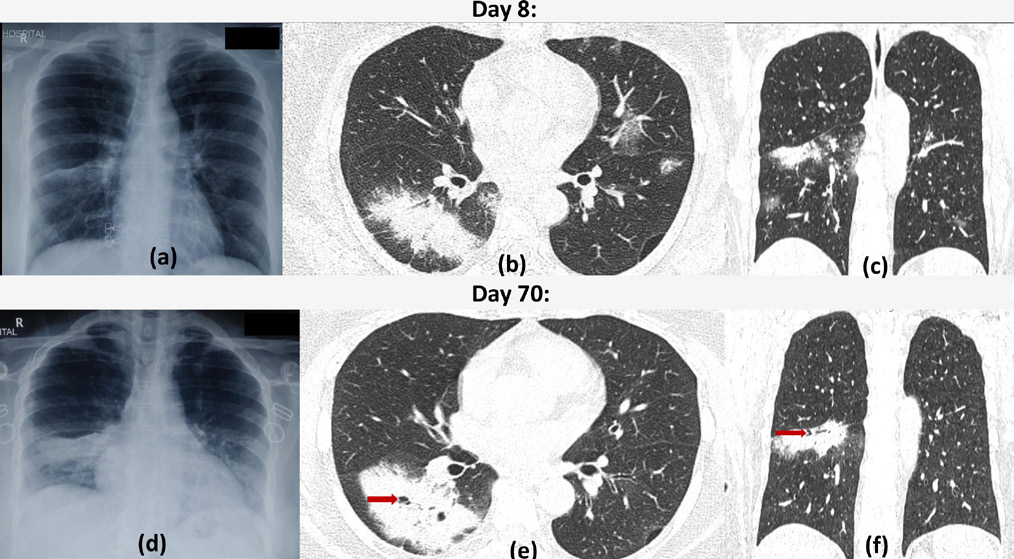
Cavitation within consolidation as a sequelae to COVID19 infection: 52-year-old female with fever tested positive for COVID19 infection, no respiratory complaints. Frontal chest radiograph (a) on the eighth day of illness shows a patchy opacity in the right mid zone. Chest HRCT (axial and coronal lung window sections - b, c) showed a patch of consolidation in the superior basal segment of right lower lobe (atypical feature of COVID19 infection) with bilateral multifocal rounded ground glass opacities. Patients symptoms progressed necessitating high flow oxygen therapy but subsequently improved and was discharged after 10 days. Follow-up frontal radiograph (d) 2 months later shows persistence of the patch. CT sections (e, f) show development of cavitation (red arrows) and non-resolution of consolidation while the ground glass opacities have resolved.
